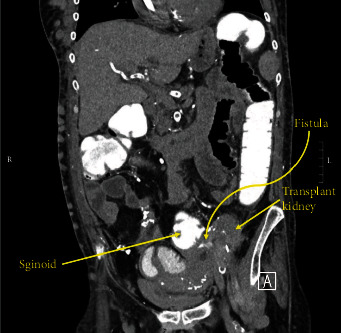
Sagittal CT of the abdomen and pelvis with rectal contrast demonstrating fistulous connection between the sigmoid colon and left renal transplant ureter.
